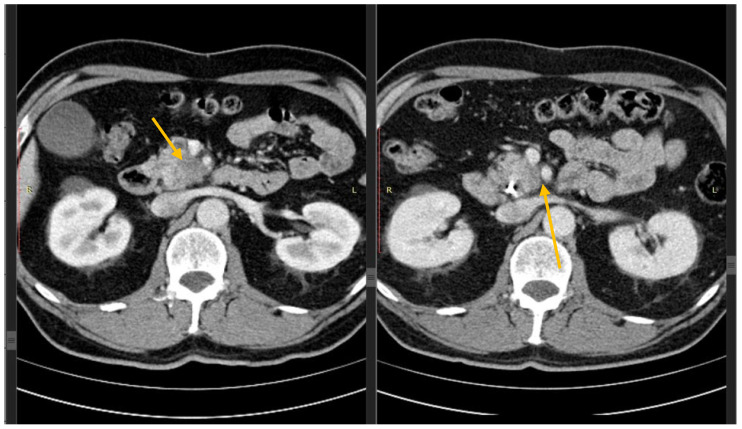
Contrast-enhanced CT shows on the left a hypovascular mass in the uncinate process (arrow), retracting the superior mesenteric vein and infiltrating the superior mesenteric artery. After chemotherapy (on the right), the mass is smaller, while a clearly visible fat plane is now seen between the lesion and the superior mesenteric artery (arrow), which represents the absolute criterion for resectability.
